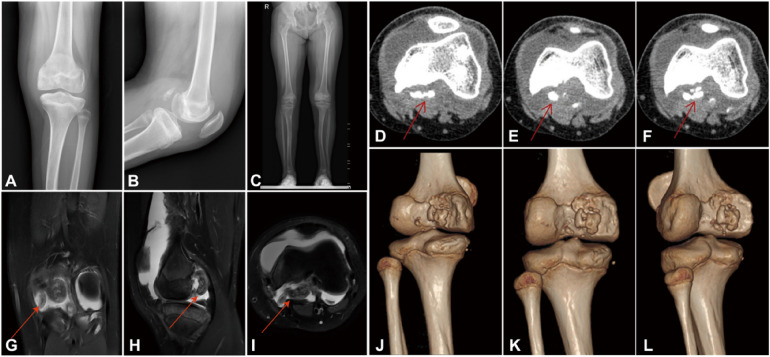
Preoperative imaging of the left knee in a 13-year-old girl with CIPA-associated charcot neuroarthropathy. (A–C) Anteroposterior (A), lateral (B), and full-length standing (C) radiographs demonstrate joint effusion, soft-tissue swelling, and irregular articular surfaces; the long-leg view provides overall coronal limb alignment. (D–F) Sequential axial CT (bone window) slices show multiple ossified intra-articular loose bodies in the posterior compartment (red arrows) with subchondral/cortical irregularities, better defining the osseous fragmentation. (G–I) Knee MRI on fluid-sensitive (PD/T2-fat-suppressed) sequences reveals massive joint effusion, synovial thickening, and extensive bone-marrow edema. Multiple low-signal intra-articular loose bodies are identified in the posterior compartment (red arrows) on coronal (G), sagittal (H), and axial (I) planes. (J–L) 3D CT reconstructions provide comprehensive visualization of osseous fragmentation/loose bodies and articular surface destruction, complementing the axial CT findings.
